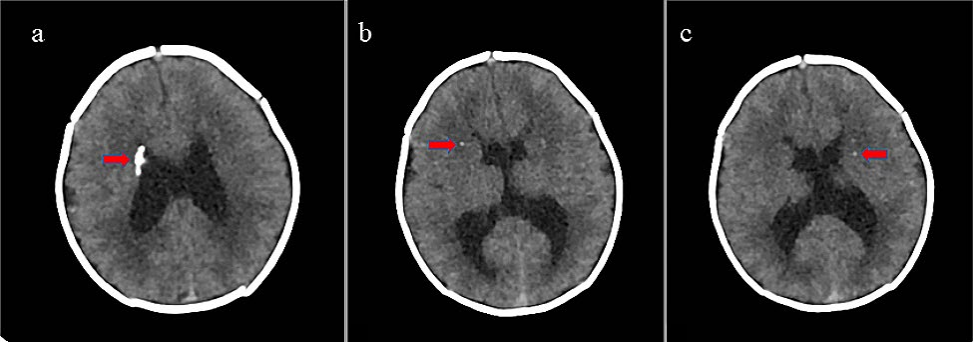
Brain computed tomography of present patient: hydrocephalus associated with subependymal calcification (a), and multiple punctate intraparenchymal hemorrhages (b and c) indicated by arrows
